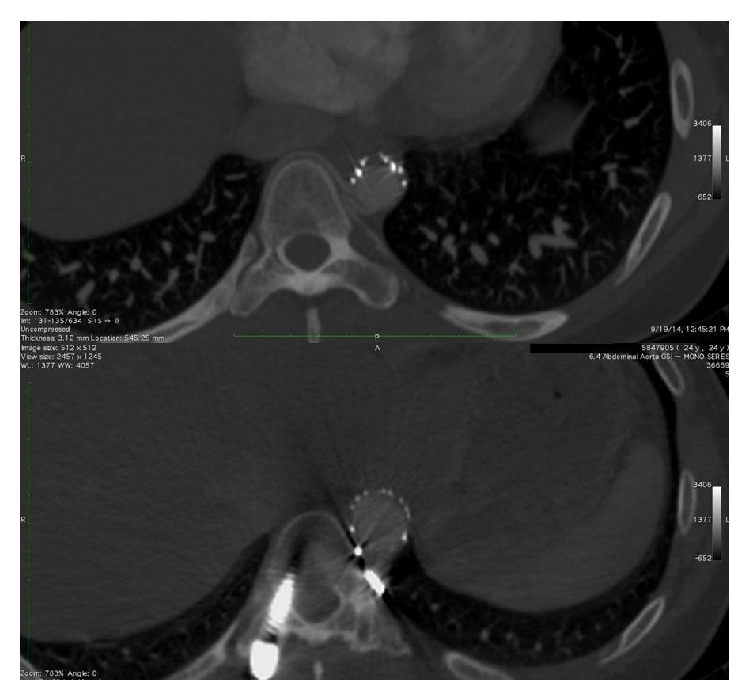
Two-year postoperative CT reveals the patency of the intercostal vessels and the restoration of the thoracic aorta.
